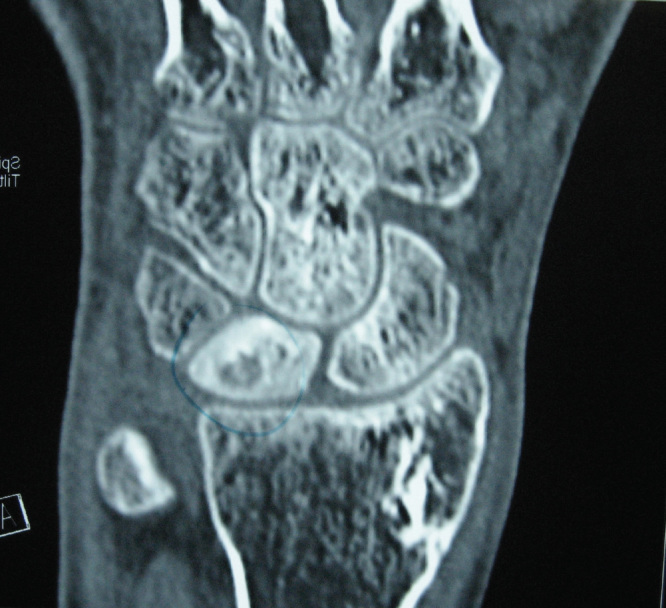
Magnetic resonance imaging view showing the patient’s wrist before first operation. Misdiagnosis had occurred due to this view in another hospital.
